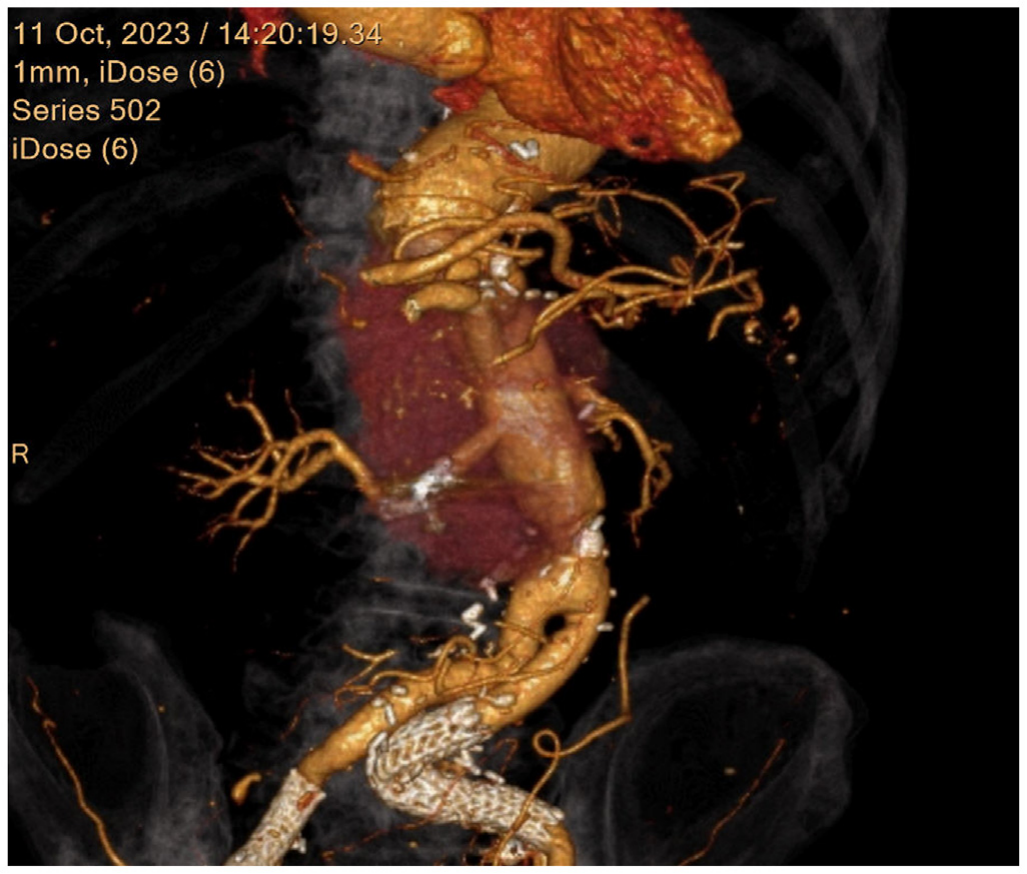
Postoperative contrast-enhanced computed tomography demonstrated no endoleak into the pseudoaneurysm sac; blood flow to the right kidney was maintained.
